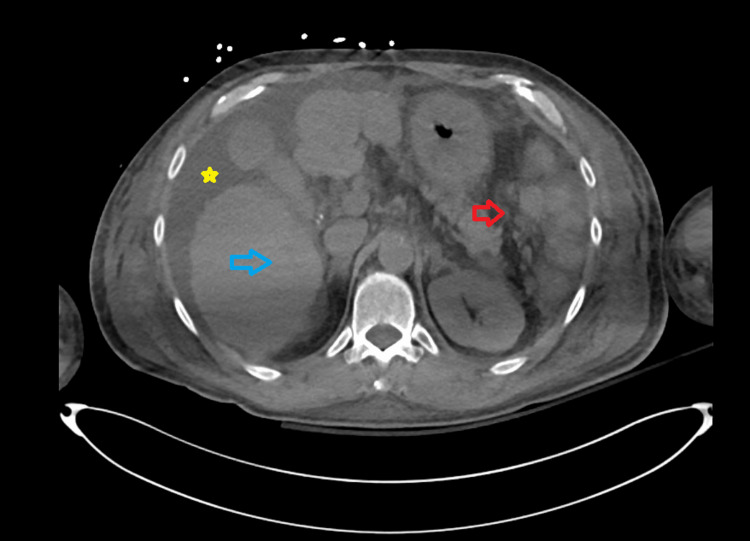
CT of the abdomen without contrast. CT of the abdomen without contrast showing cirrhosis (blue arrow), ascites (yellow star), and absent spleen (red arrow).
